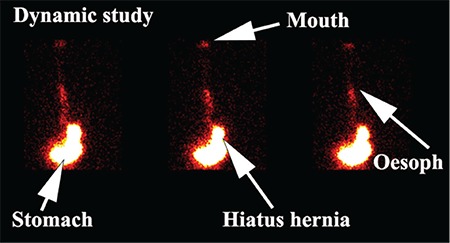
Dynamic scintigraphic study. The sequence of images demonstrates tracer activity in the stomach with evidence of a hiatus hernia and gastro-oesophageal reflux to the level of the oropharynx. Note the progressive accumulation of tracer in the region of the oropharynx (mouth)
